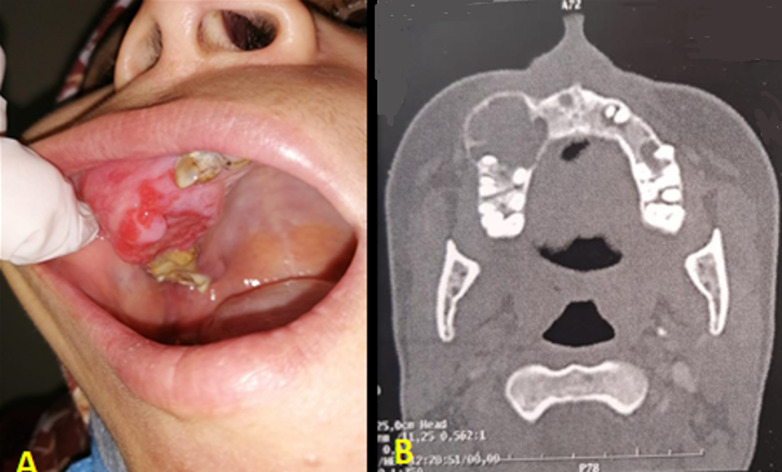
endobuccal view showing swelling of the upper right molar region occupying the corresponding vestibule area with inflammatory mucosa (A); computed tomography on axial view showing an osteolytic image of the right maxilla crossed by septas and causing thinning of the cortical (B)
